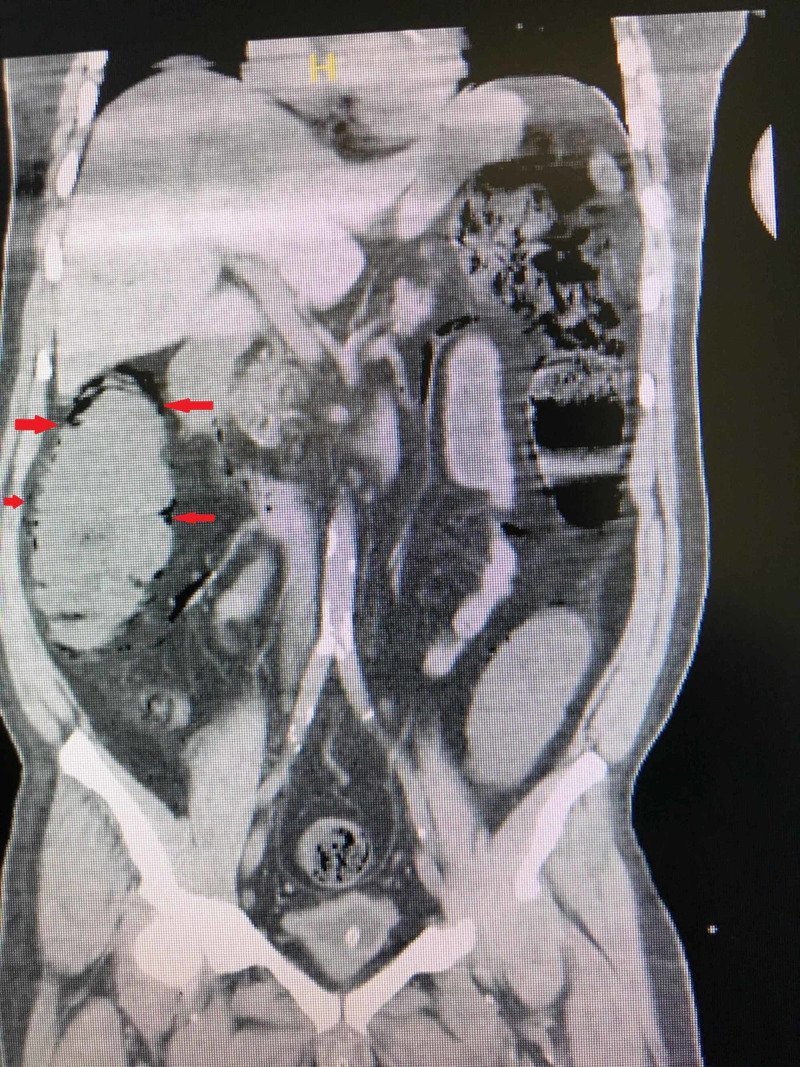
CT scan, coronal plane Red arrows are showing the pneumatosis cystoides intestinalis in the bowel wall
